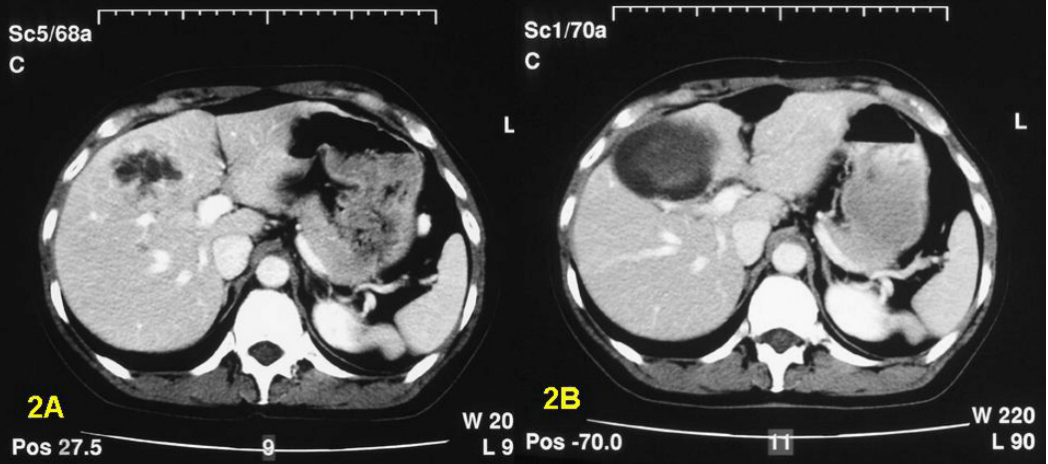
This slide of the CT-scan of patient 14 shows one dominant metastasis in the right liver lobe, but several other small nodules are present (figure 2A). After RFA necrosis with typical cystic appearance developed (figure 2B).
